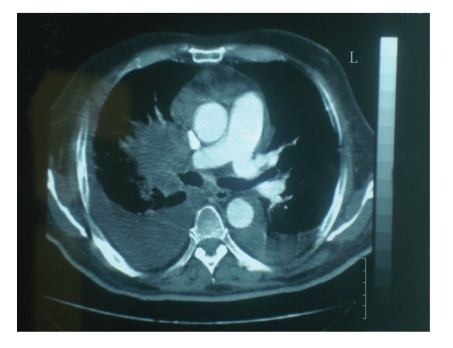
CT arteriography at the carina showing occlusion of the right pulmonary artery due to external compression from a mass of 6.8 cm and atelectasis. No intraarterial thrombus is seen. Left pulmonary artery is nearly completely occluded from lymph node mass of 2.3 cm. Extensive mediastinal lymphadenopathy was also seen. Pleural effusions are also evident.
